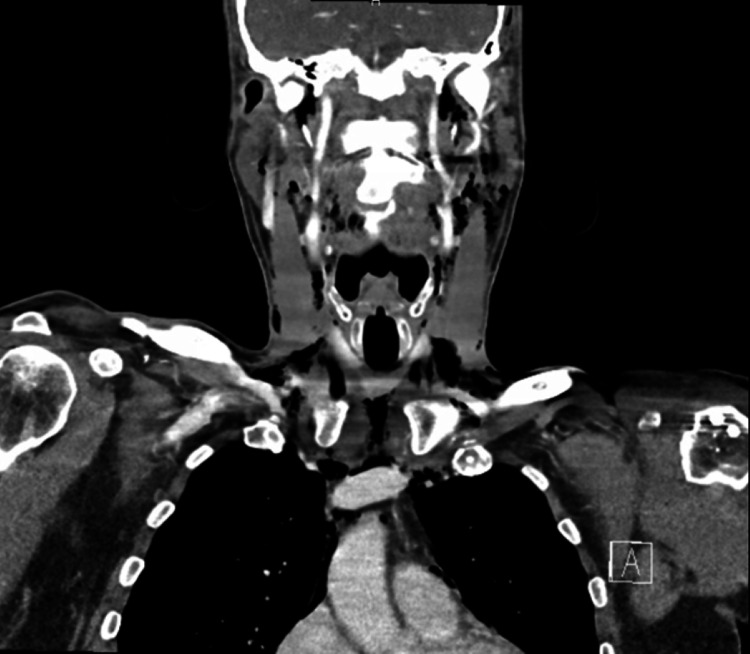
Contrast-enhanced CT scan of the neck and thoracic area showing extensive subcutaneous emphysema CT: computed tomography
